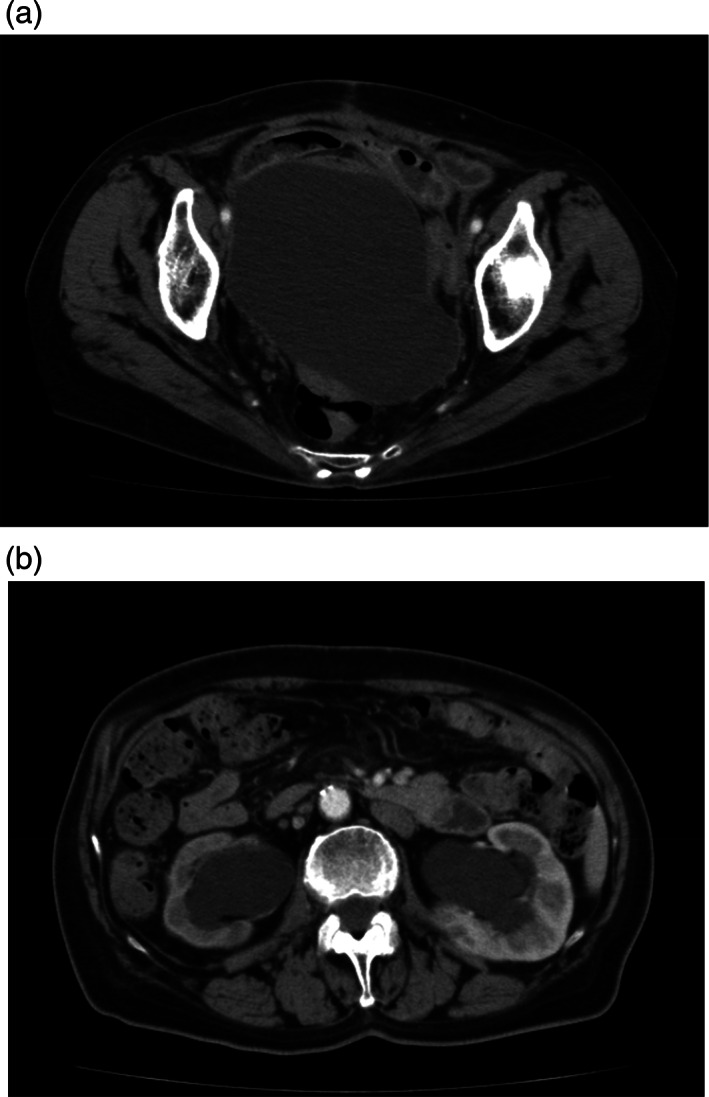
Three months after laparotomy including pelvic lymph node dissection, a large pelvic lymphocele (a) and bilateral hydronephrosis (b) were detected by computed tomography
